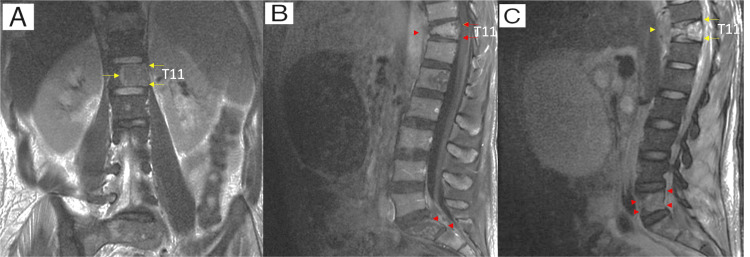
Neuroaxis MRI images Neuroaxis MRI showing multiple areas of osteolysis in the bone marrow of the T11 vertebral body (A and C: yellow arrows, B: red arrows); the sacral parts and the iliac bones (B and C: red arrows head). It is associated with a reduction in the height of the anterior aspect of the T11 vertebral body by more than 90% and a collection with peripheral contrast enhancement that extends to the pre-and paravertebral soft tissues at the T11 level (B and C: red and yellow arrows head). The imaging pattern is not specific but allows us to consider an association with the clinical suspicion of inflammatory/infectious involvement. Neoplastic nature is less likely, although it cannot be completely ruled out.
